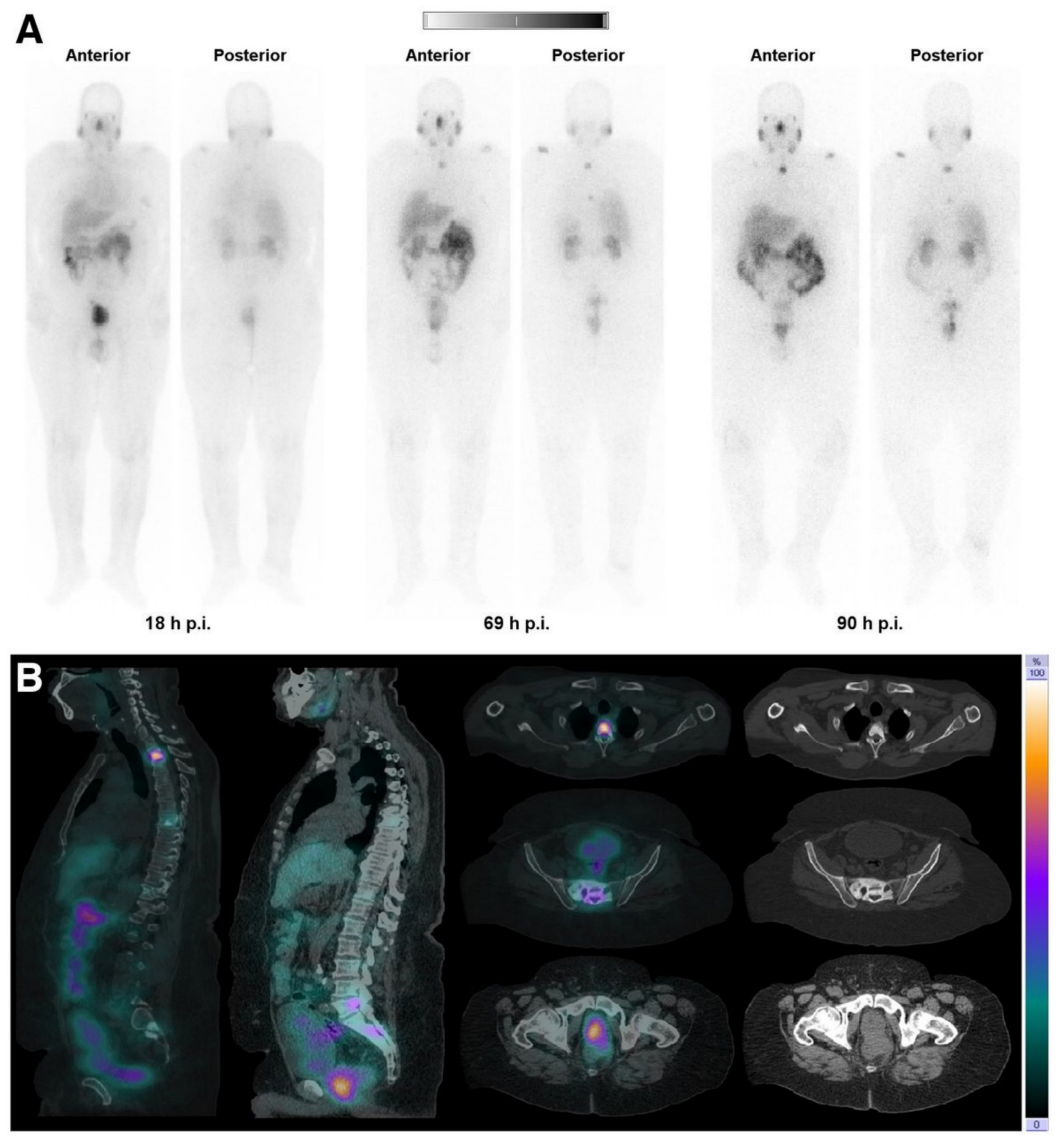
(A) Whole-body images at different time points after injection of 5550 MBq of [^161^Tb]Tb-PSMA-617. (B) Representative SPECT/CT sagittal and axial slices and CT axial slices demonstrating physiologic biodistribution in lacrimal, parotid, and submandibular glands; nasopharyngeal mucosa; liver; intestinal tract; kidneys; and urinary bladder, as well as pathologic uptake in primary prostate tumor and metastatic bone lesions. (p.i. = post-injection). Reprinted with permission from Al-Ibraheem *et al*. 96, copyright 2023 by the Society of Nuclear Medicine and Molecular Imaging.
